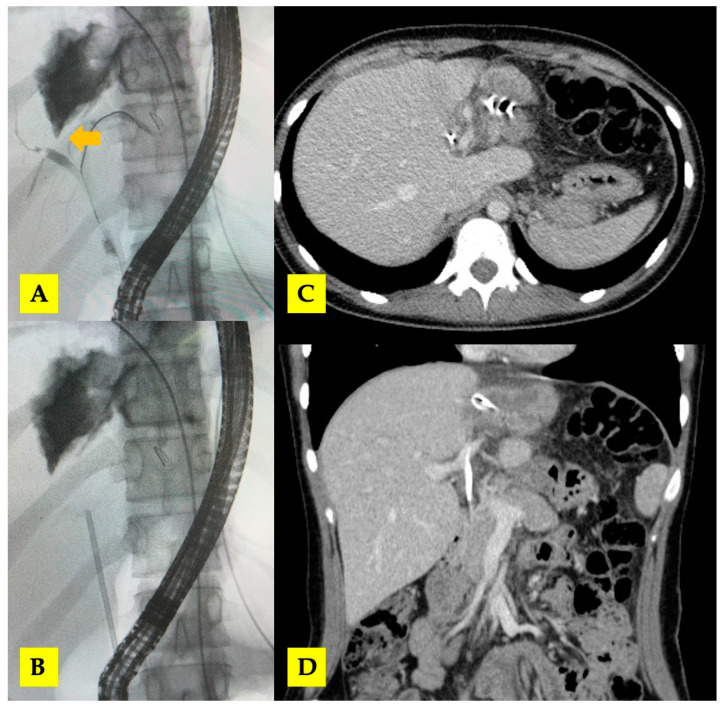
(A,B) ERCP with biliary stent placement (arrow—central biliary communication with right hepatic duct); (C,D) post-ERCP CT scan with biliary stent in situ.
